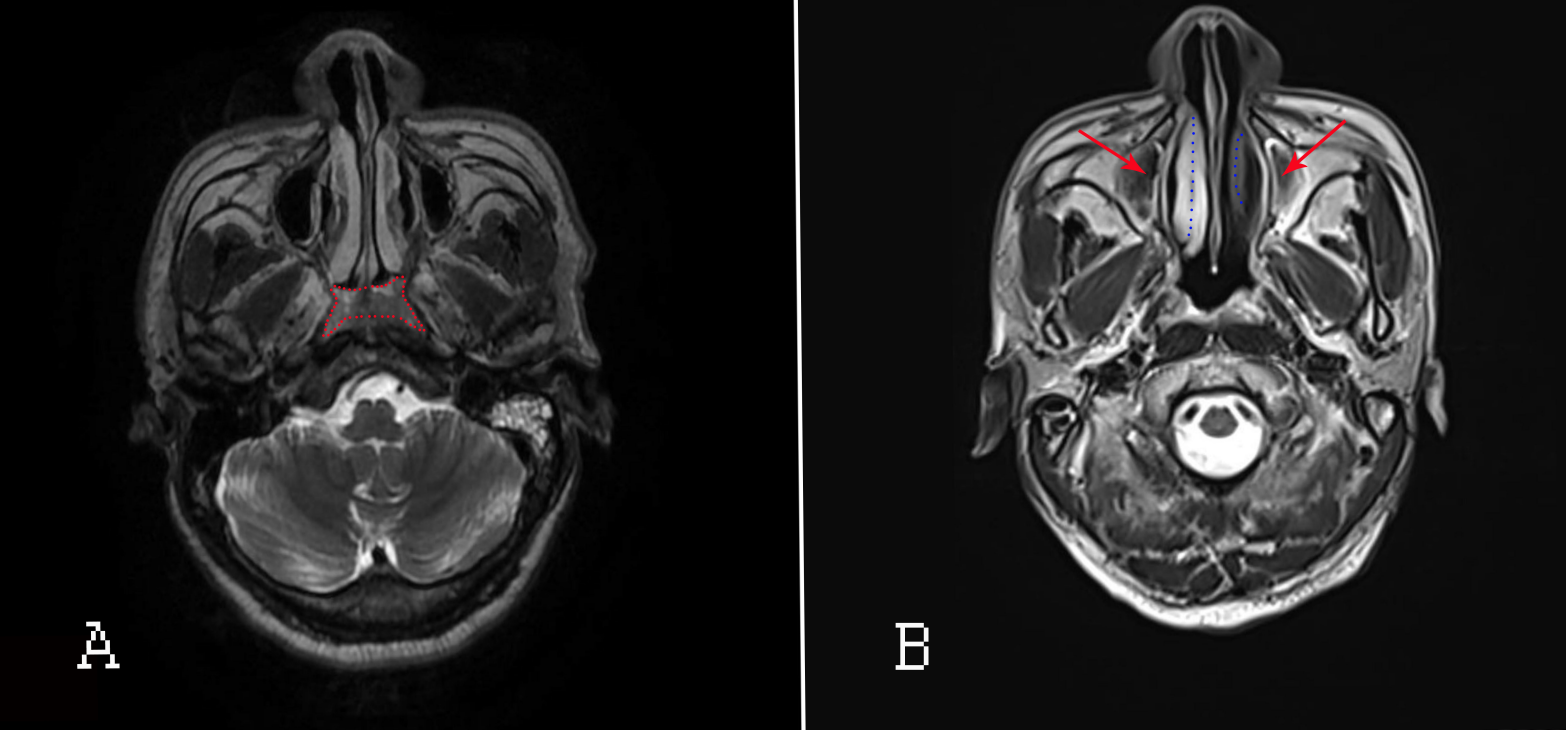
MRI of the nasopharyngeal passages and skull base. (A) Before RT; the red area indicates nasopharyngeal lesions. (B) After RT. Red arrows: inflammation of the maxillary sinus; blue dotted lines: oedematous IT (right) and atrophied IT (left).
